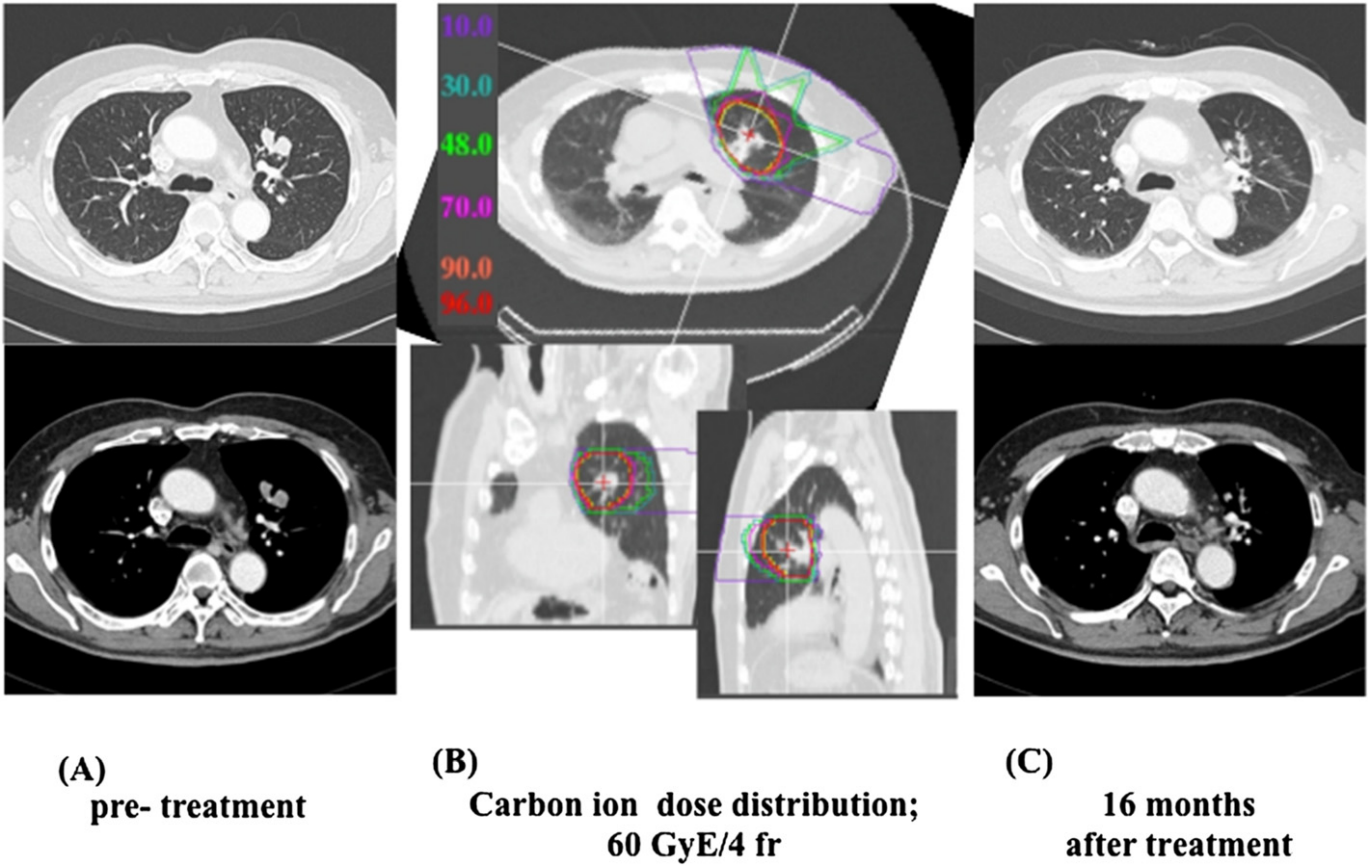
Carbon ion radiotherapy and radiographic changes in a typical patient. (A) Pre-treatment CT scan of oligo-recurrence in the lung from colorectal cancer. (B) Radiotherapy plan for oligo-recurrent pulmonary lesion, which received 60 GyE in four fractions. The yellow line indicates the clinical target volume that was delineated in the expiratory phase. Dose distribution is shown with isodose lines of different colors (color scale on left side of image). The clinical target volume is fully encompassed by the 95% isodose line. (C) Chest CT scan 16 months after irradiation, revealing no evidence of recurrence.
